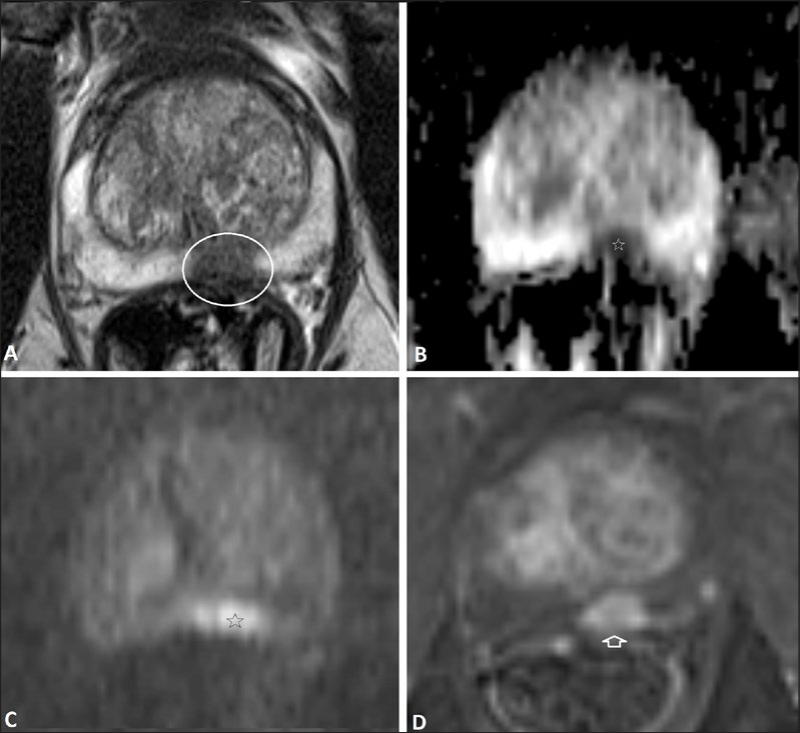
60-year-old men with elevated PSA (20 ng/ml). On axial T2-weighted image (A) A nodular lesion with low SI is noted (white circle) posteriorly in de peripheral zone of the mid prostate. This nodule shows low ADC value (B) and High SI on high-b-value image (C) of DWI, and strong Gadolinium contrast enhancement (D), suspicious for a high-grade prostate cancer, PI-RADS overall assessment score 5. A targeted transrectal ultrasound guided biopsy was performed, confirming a Gleason 4 + 4 prostate cancer.
